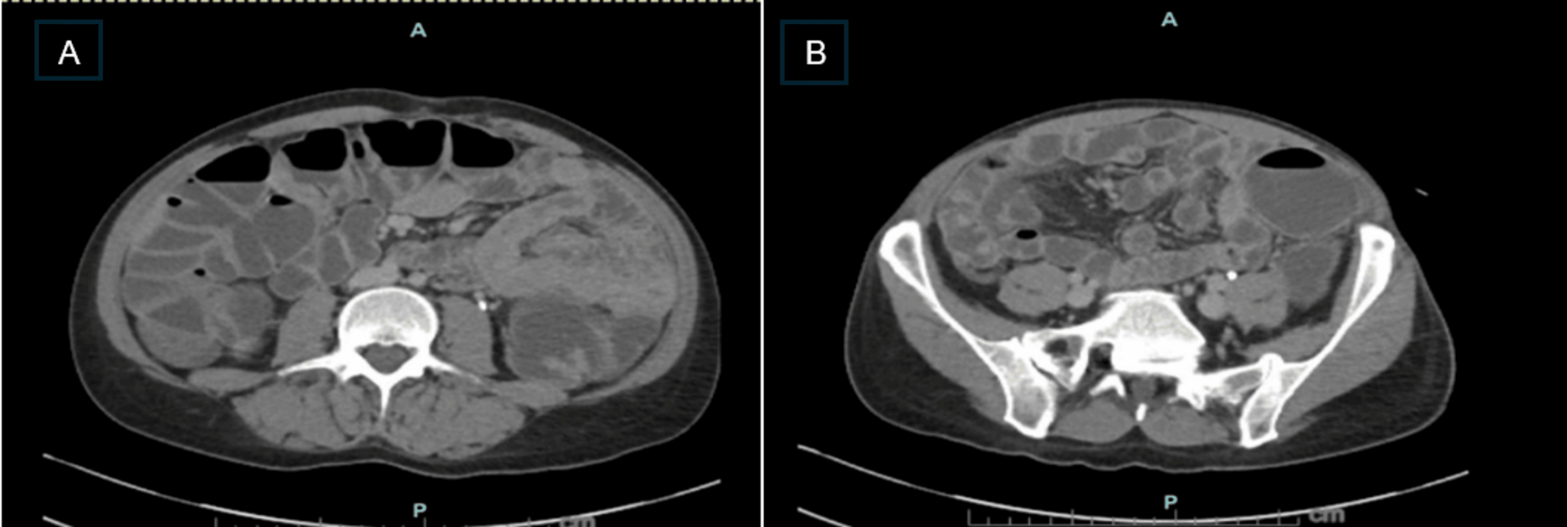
CT abdomen/pelvis imaging on presentation Axial CT images of the abdomen showing a fluid-filled, distended colon (A) and small bowel loops (B).
